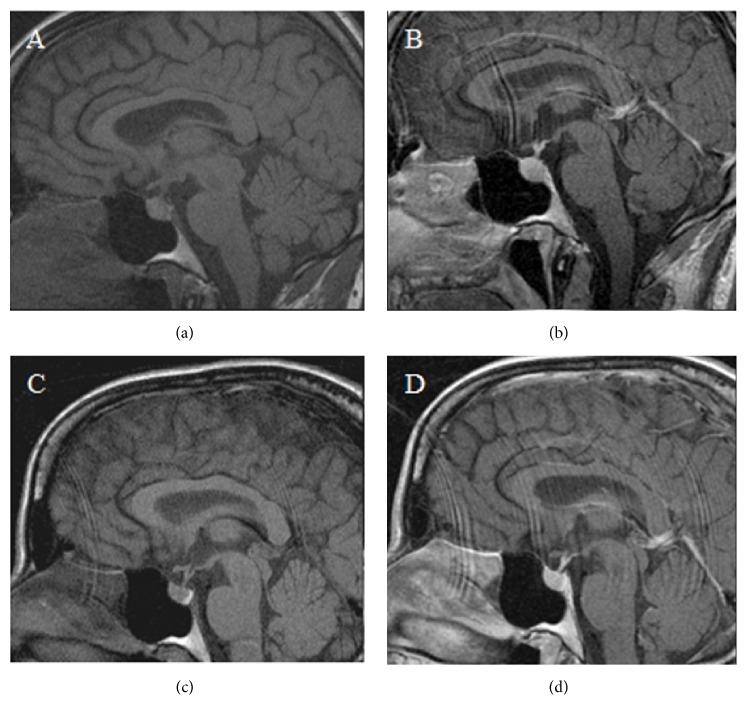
(a, b) Pre- and postcontrast brain MRI images of case 1 show that posterior pituitary bright spot is not visualized. (c, d) Pre- and postcontrast brain MRI images of case 1 at four months postpartum show return of posterior pituitary bright spot.
